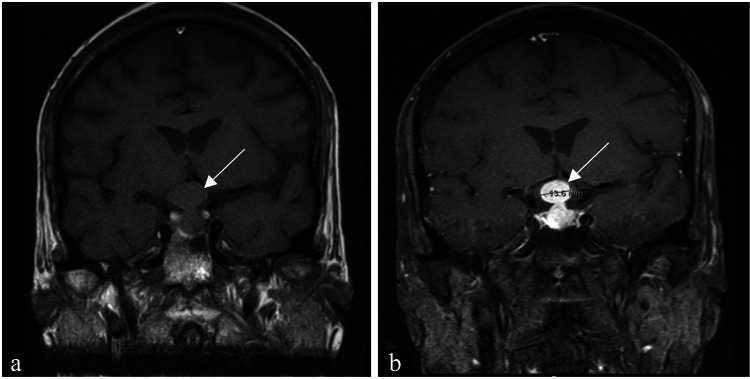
MRI scan (a) MRI scan of the pituitary gland showing an iso-intense dumbbell-shaped lesion (arrow) with thickening of the stalk. (b) Homogenous enhancement of the lesion (arrow) after contrast. MRI, magnetic resonance imaging
